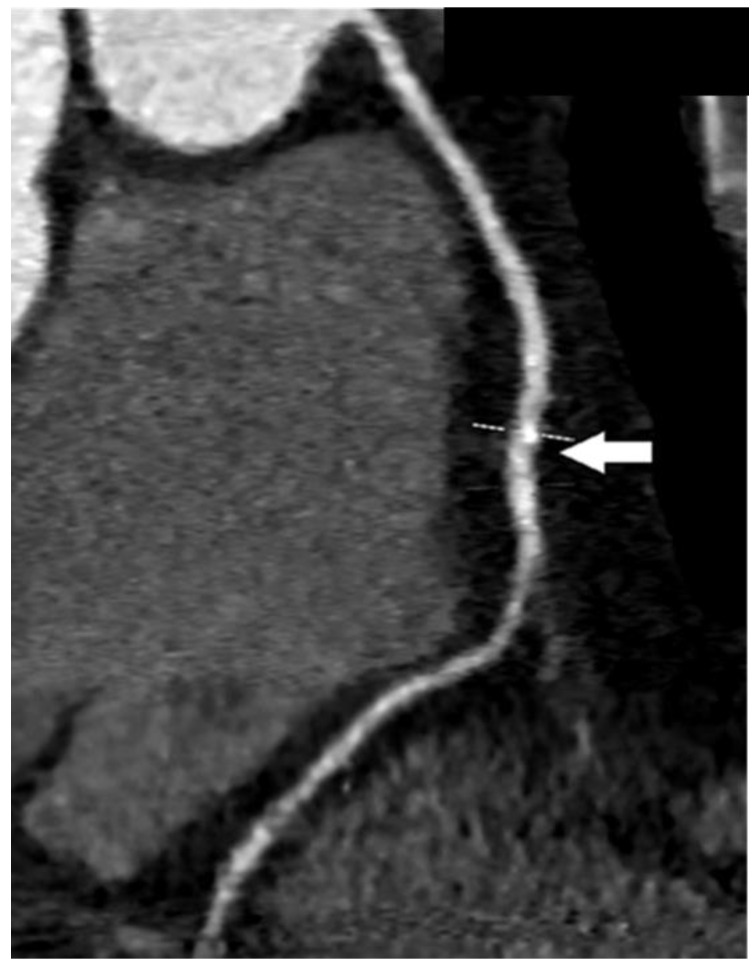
Coronary computed tomographic angiography (CCTA), oblique view, maximum intensity projection (MIP). The right coronary artery is presented with the normal course and lumen width. The white arrow shows a non-obstructive calcification of the artery wall. Copyright: the authors.
